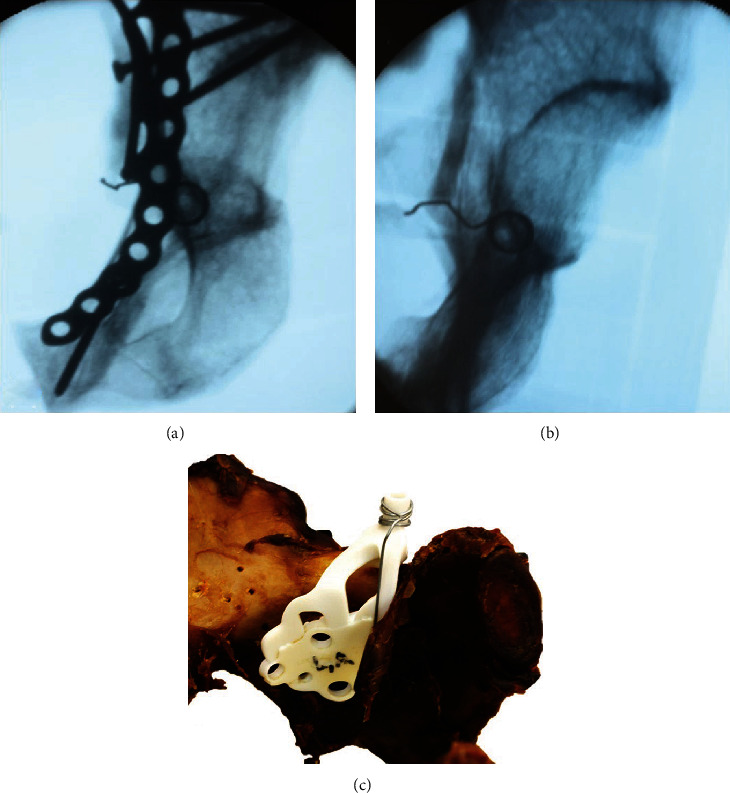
Fluoroscopy image for fine adjustment of the plate position (a) and an image showing the relation of Koehler's teardrop (center of the metal ring) and the drill guide (b) in a combined inlet oblique view along the infra-acetabular canal. Drill guide with a radiographic marker on a left acetabulum (c).
